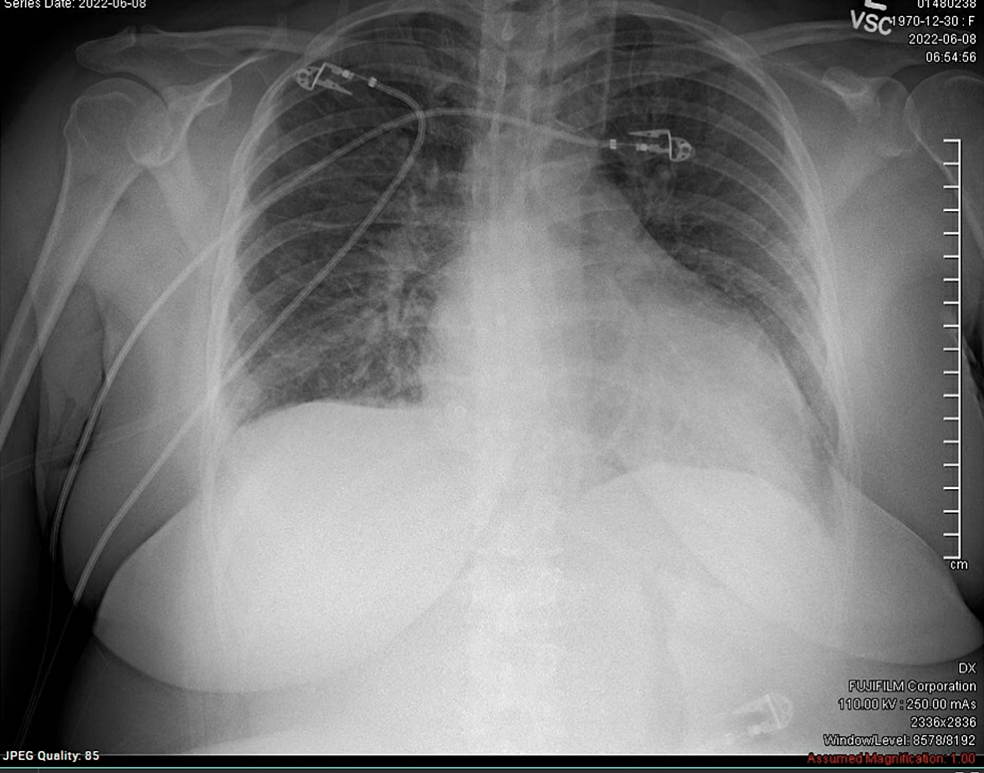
AP chest X-ray on arrival at the emergency department The X-ray was read as mild right basilar atelectasis with possible vascular congestion
